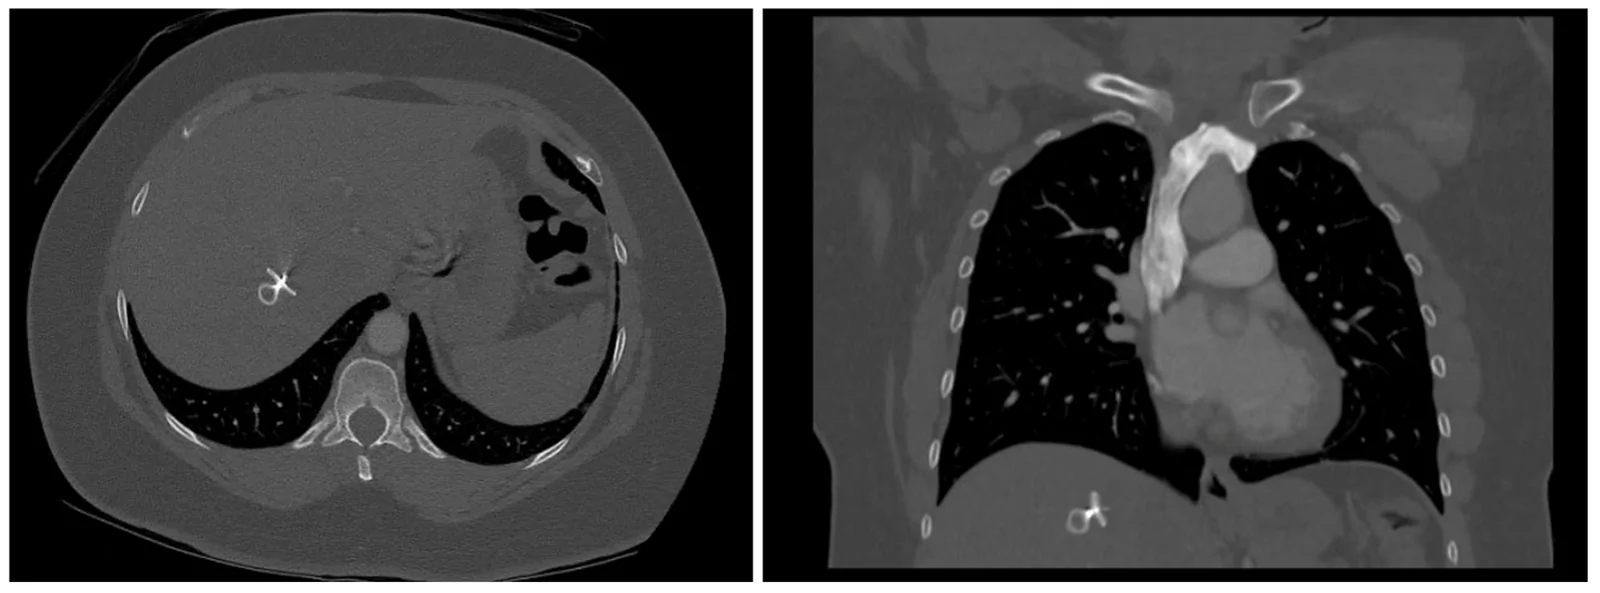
CT performed 20 days after surgery showing presence of vascular plugs in the liver.
